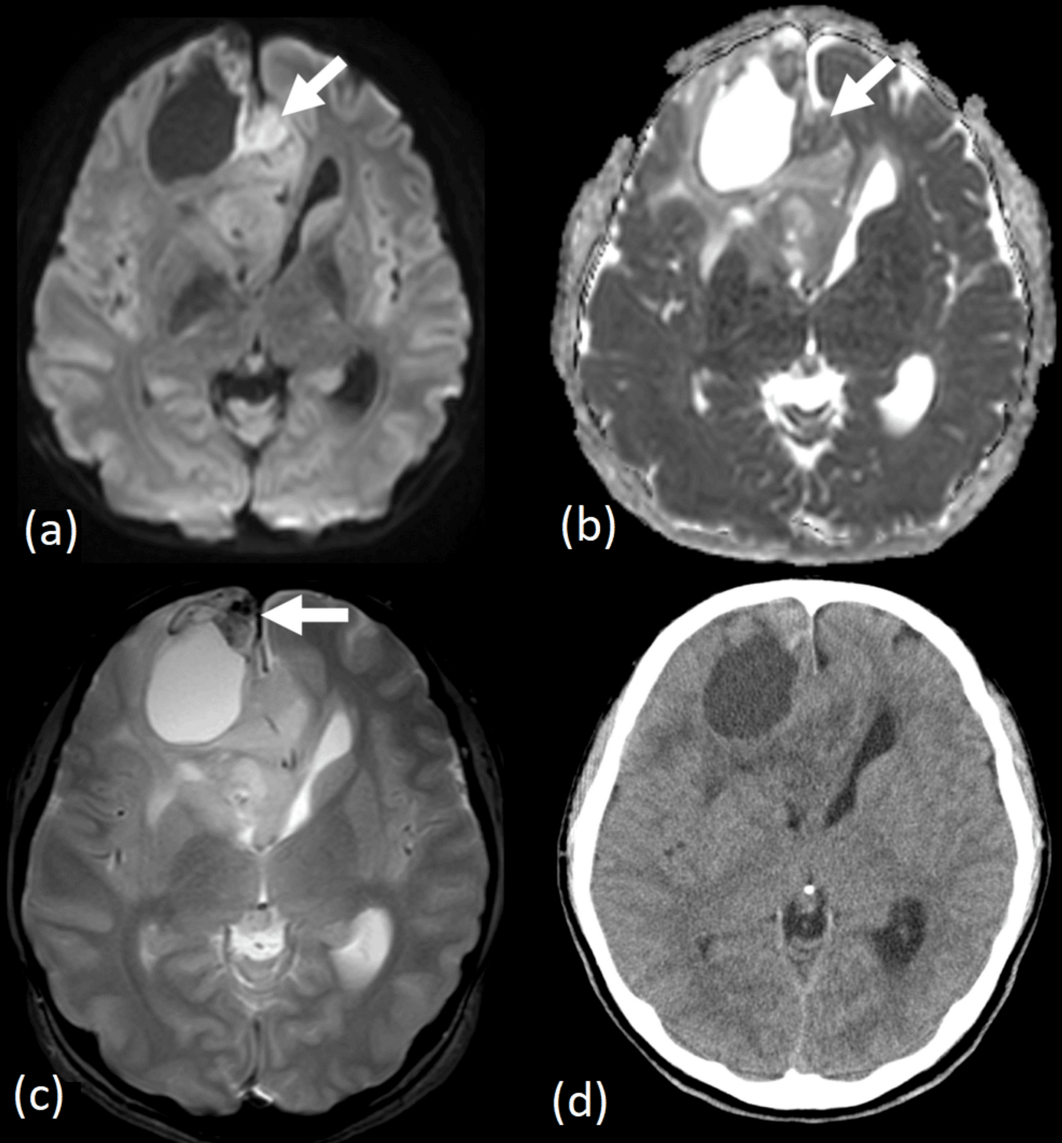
CT and MRI images Diffusion-weighted imaging shows restricted diffusion within the enhancing region (a; arrow), with a minimum apparent diffusion coefficient of 0.73 × 10⁻³ mm²/s (b; arrow). T2*-weighted imaging reveals a hypointense area in the anteromedial portion of the tumor, suggestive of intratumoral hemorrhage (c; arrow). Non-contrast CT demonstrates a 37-mm lesion, with necrotic components but no obvious calcification (d). MRI, Magnetic resonance imaging; CT, Computed tomography
